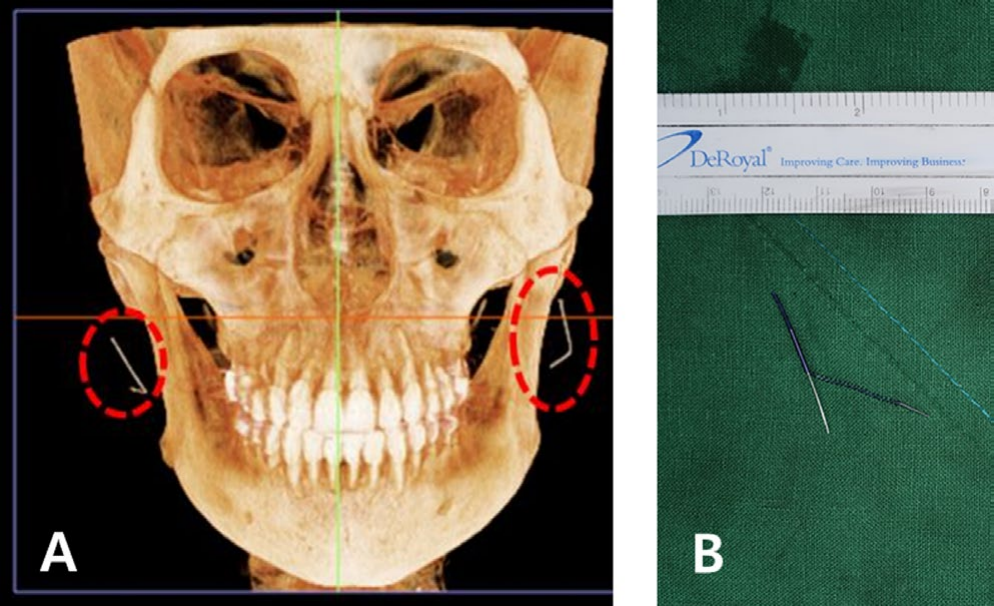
When using cannula‐integrated threads, there is a possibility that the cannula and hub (handle part) can separate, leaving the cannula embedded inside. Shown in a computed tomography image (A) and during cannula removal (B).
